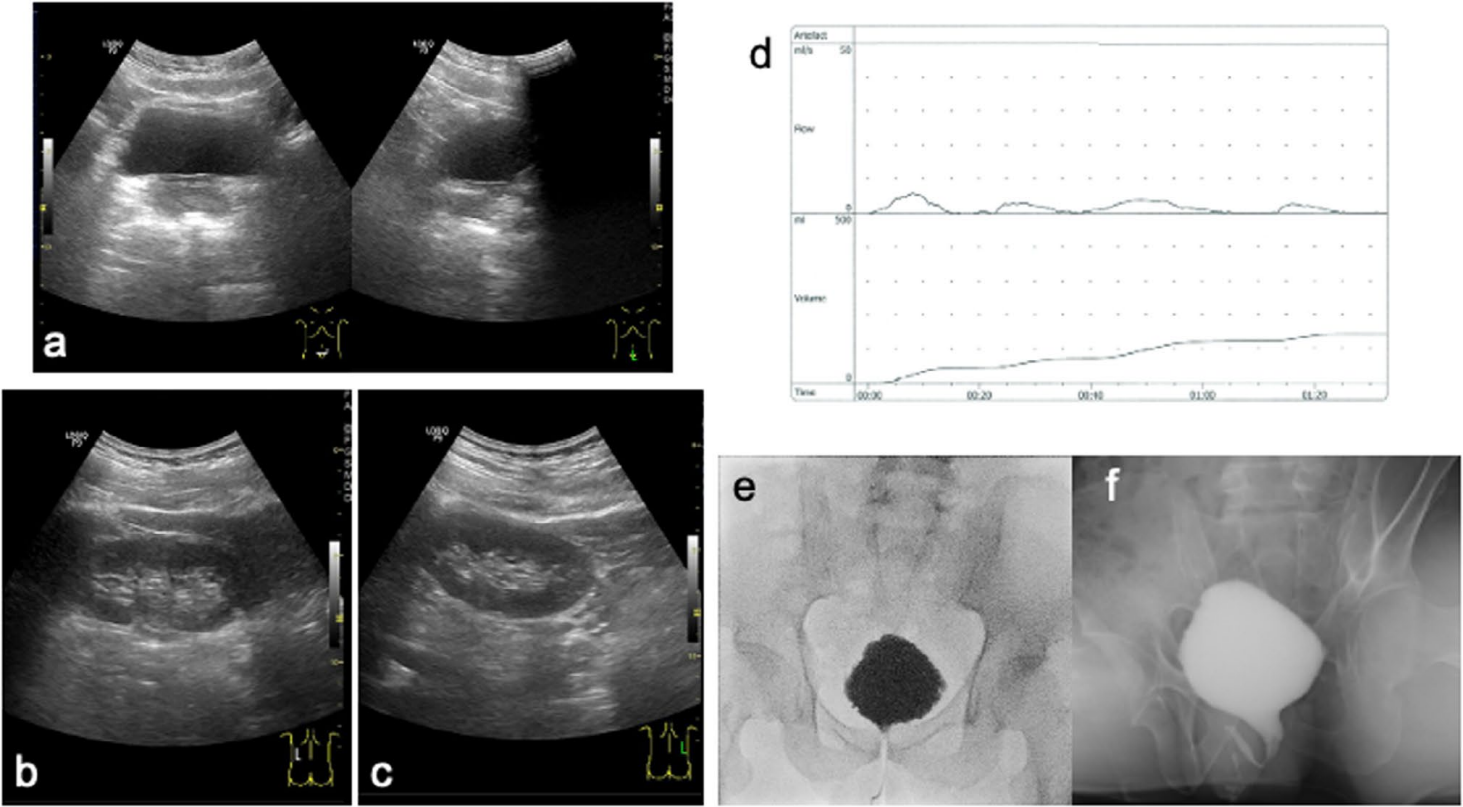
Abdominal ultrasonography (a–c), uroflowmetry (d), and VCUG (e, f). (a) Bladder. No bladder deformity is apparent. (b) Right kidney; (c) left kidney. No dilatation of either upper urinary tract is evident. (d) Findings from uroflowmetry. Voided volume, 142 mL: Residual urine volume, 550 mL; maximum flow rate, 5.9 mL/s; flow pattern, interrupted. (e) VCUG in storage phase. Bladder deformity is revealed, VUR is not present. (f) VCUG in voiding phase. Relaxation of the urethral sphincter is inadequate.
